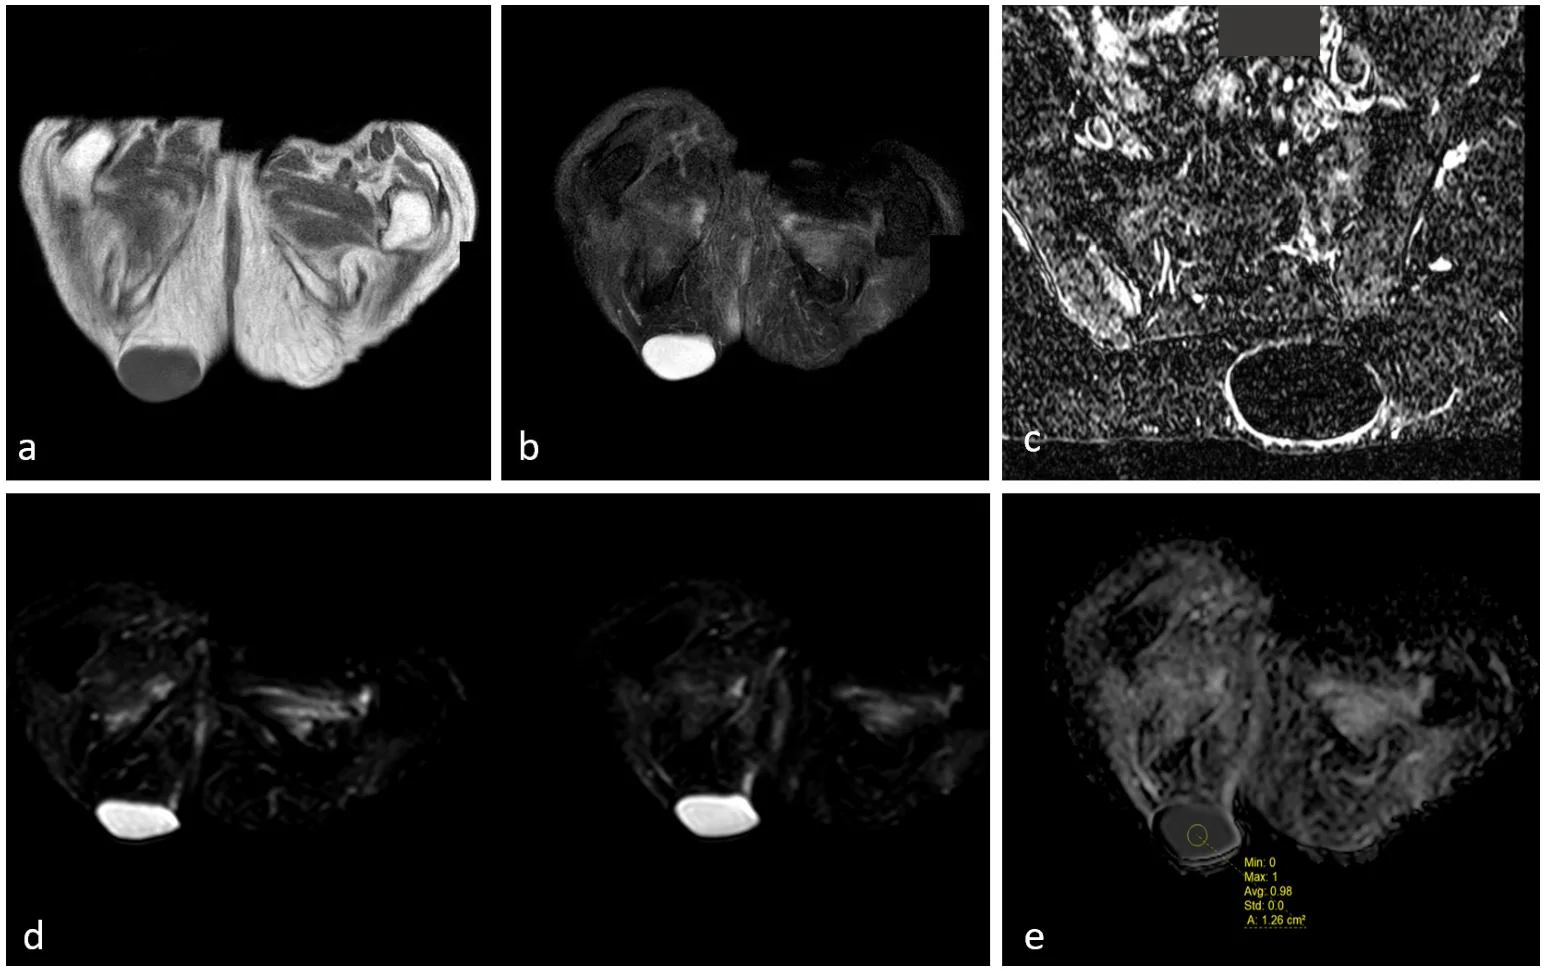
Axial MRI of an epidermoid cyst demonstrating T1-weighted hypointense (a) and T2-weighted hyperintense (b) signal with thin peripheral enhancement on subtracted post-contrast T1-weighted imaging (c). Despite its benign nature, the lesion shows high signal on DWI (d), with corresponding restricted diffusion on the ADC map (e), representing a potential diagnostic pitfall. This appearance is attributed to the viscous internal contents of the cyst, including keratin, cholesterol, and proteinaceous debris, which impede free water diffusion and mimic the restricted diffusion pattern of aggressive or malignant lesions.
